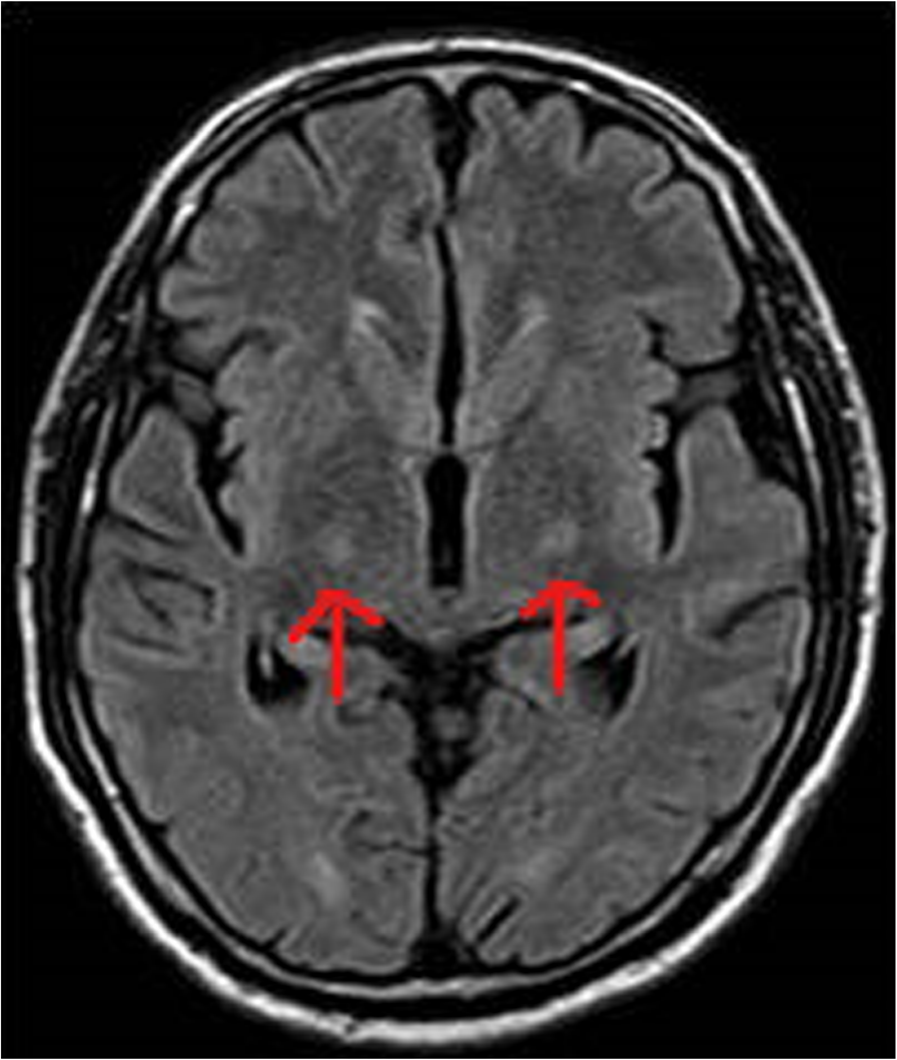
Axial FLAIR sequence at the level of ganglio-thalamic region showing hyperintensity in posterior limbs of bilateral internal capsules (red arrows)
